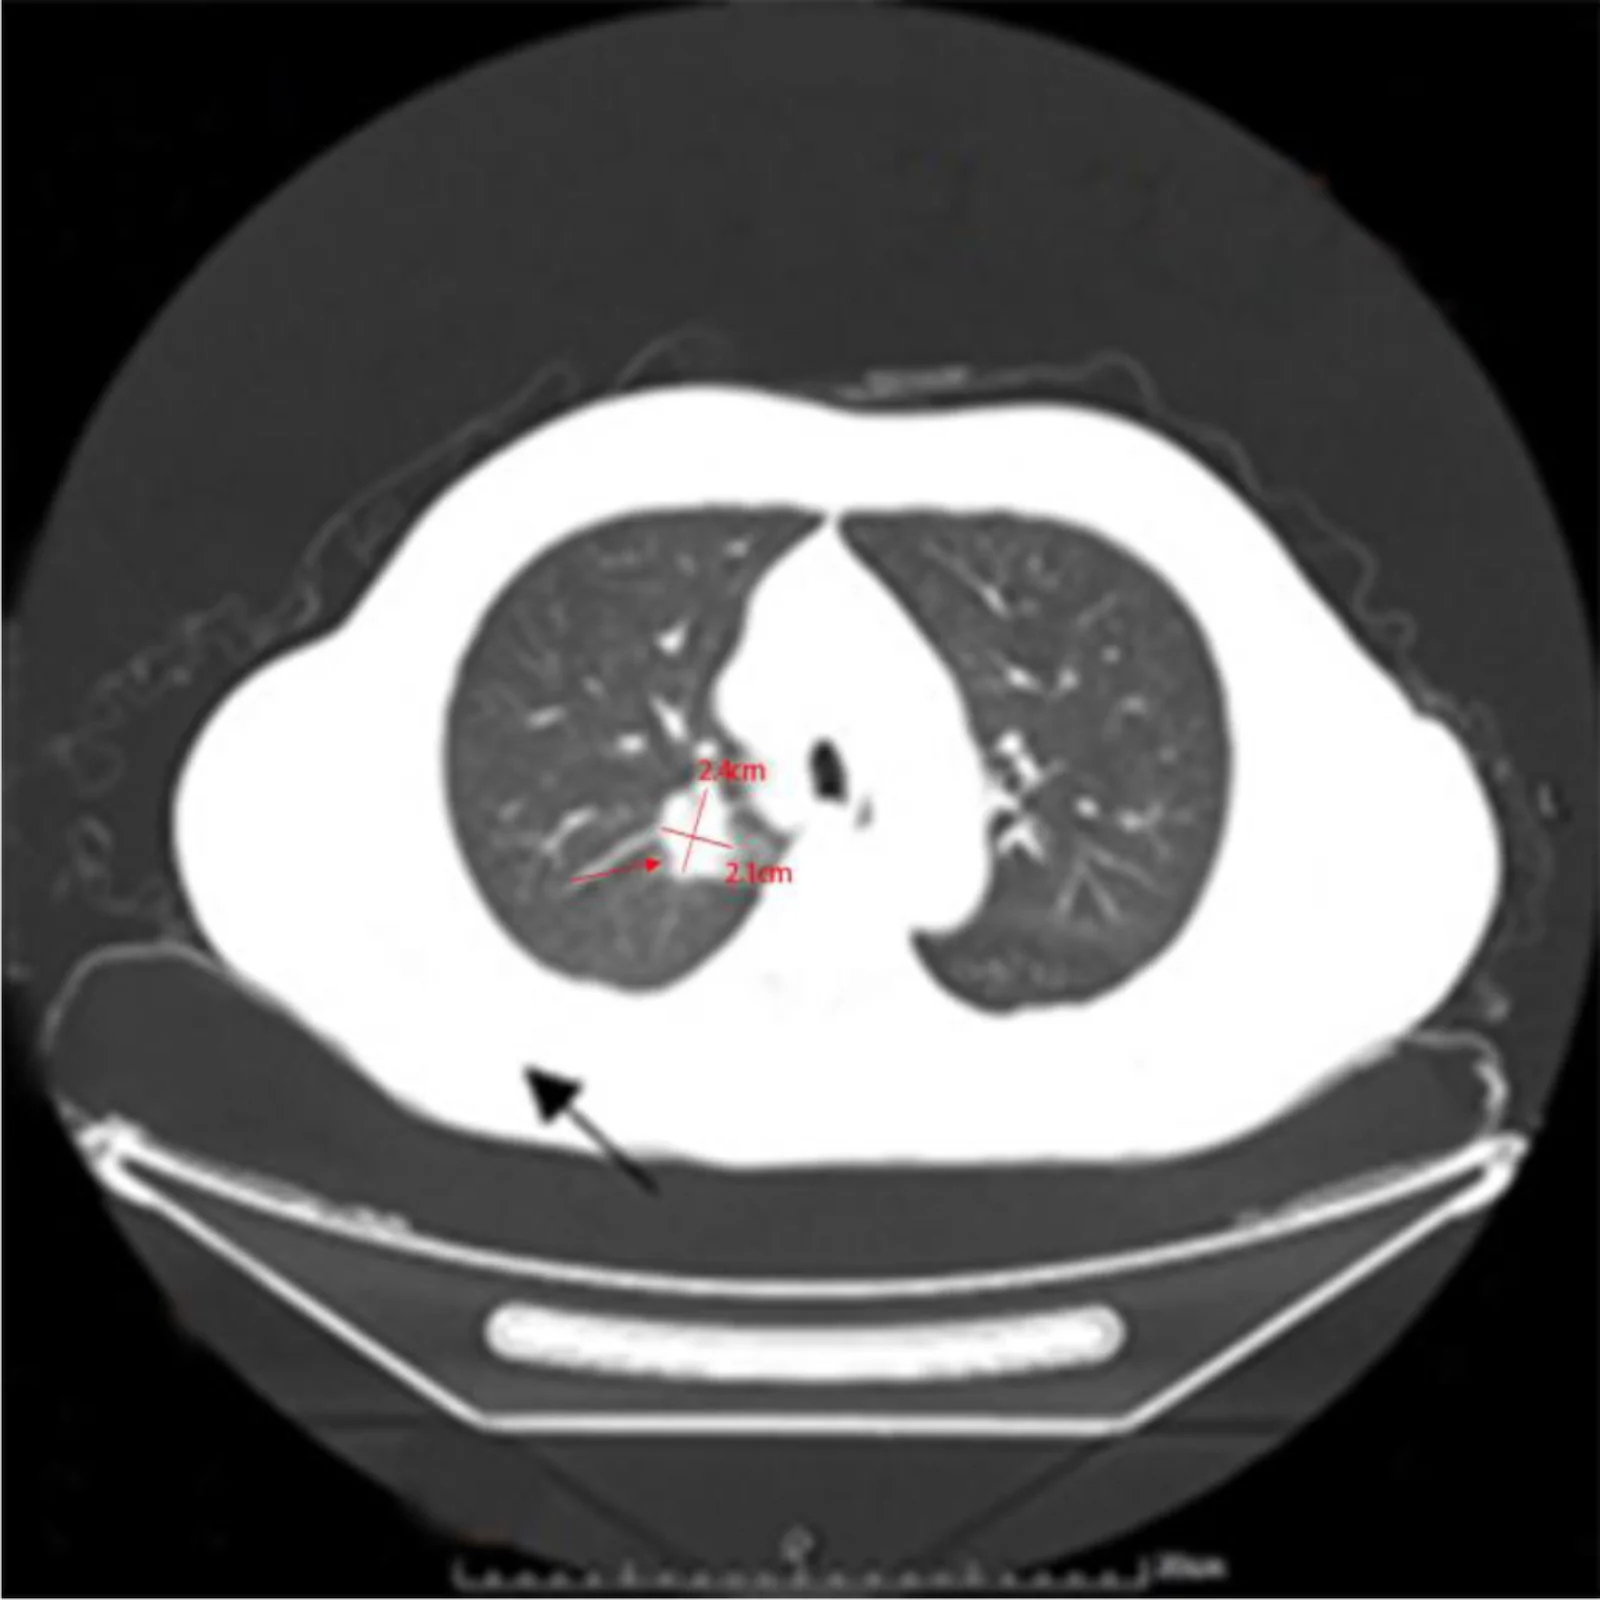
June 2020, lung window and mediastinal of CT scan showed a 2.1 × 2.4 cm right pleural. CT = computed tomography.
